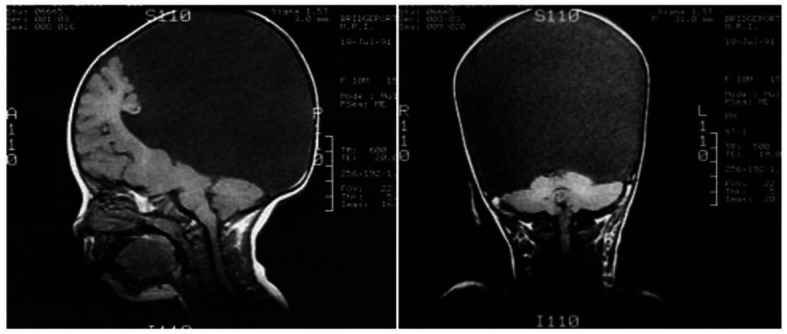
Congenitally decorticate children MRI brain scan (midline sagittal and posterior coronal plane). Credit: Shewmon et al. (1999). Reproduced with permission from Wiley.
